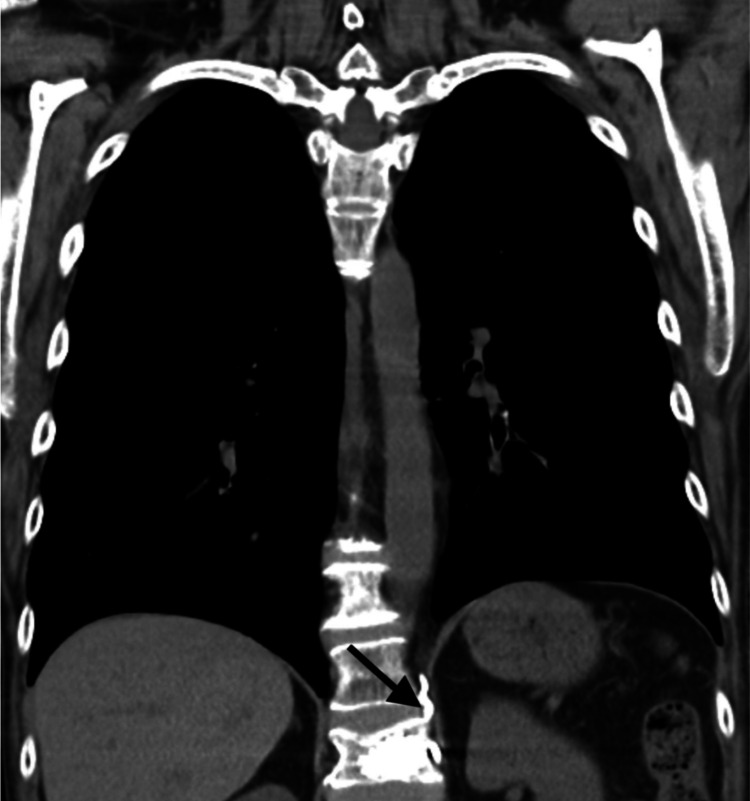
CT showing extravasation of polymethylmethacrylate (PMMA) in paravertebral veins as indicated by the arrow.
